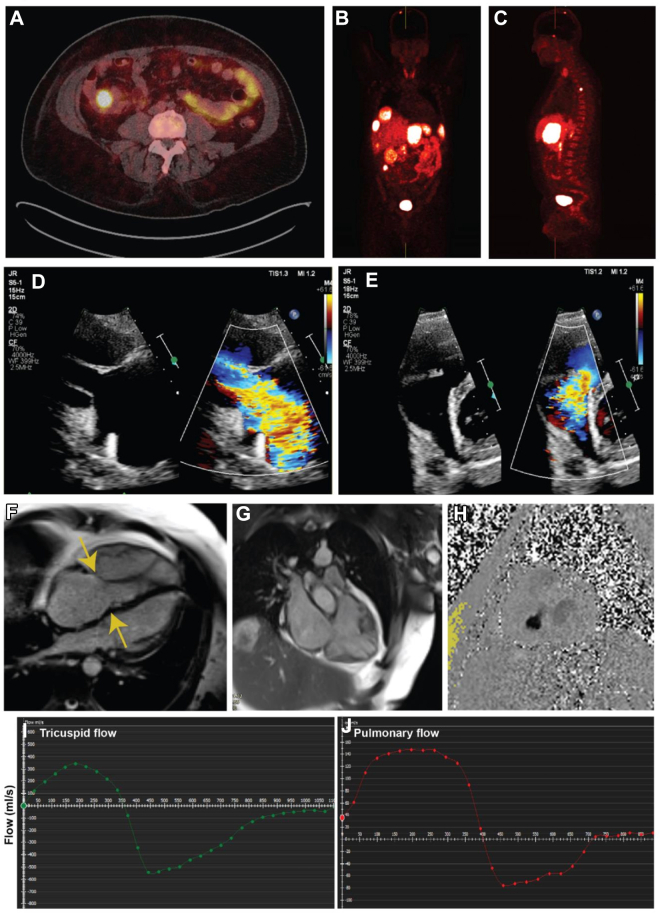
Baseline Characterization of Lesions and Cardiac State (A to C) ^68^Ga-Dotatate PET/CT demonstrating somatostatin positive lesions on axial, coronal, and sagittal planes, respectively. (D) Preoperative TTE demonstrating severe TR (TR jet area: 19 cm^2^, vena contract 23 mm), RA dilation (RA ESA Index 18.7 cm^2^/m^2^) and RV dilation (RV basal diameter 5.1 cm, mid-diameter 4.5 cm, diastolic area 34.4 cm^2^) with thickened and retracted valve leaflets. (E) Preoperative TTE demonstrating a thickened pulmonary valve with mild pulmonary stenosis and regurgitation. (F and G) CMR demonstrating thickening, shortening, and retraction of the tricuspid valve leaflets (F), resulting in a large coaptation defect with severe TR (G). (H) CMR demonstrating thickening of the PV cusps with moderate-severe PV stenosis (valve area 1.0-1.1 cm^2^, peak velocity 2.3 m/s). (I and J) CMR-derived tricuspid and pulmonary transvalve flow velocity curves. CMR = cardiovascular magnetic resonance; ESA = end systolic area; PET/CT = positron emission tomography/computed tomography; PV = pulmonary valve; RA = right atria; RV = right ventricle; TR = tricuspid regurgitation; TTE = transthoracic echocardiography.
